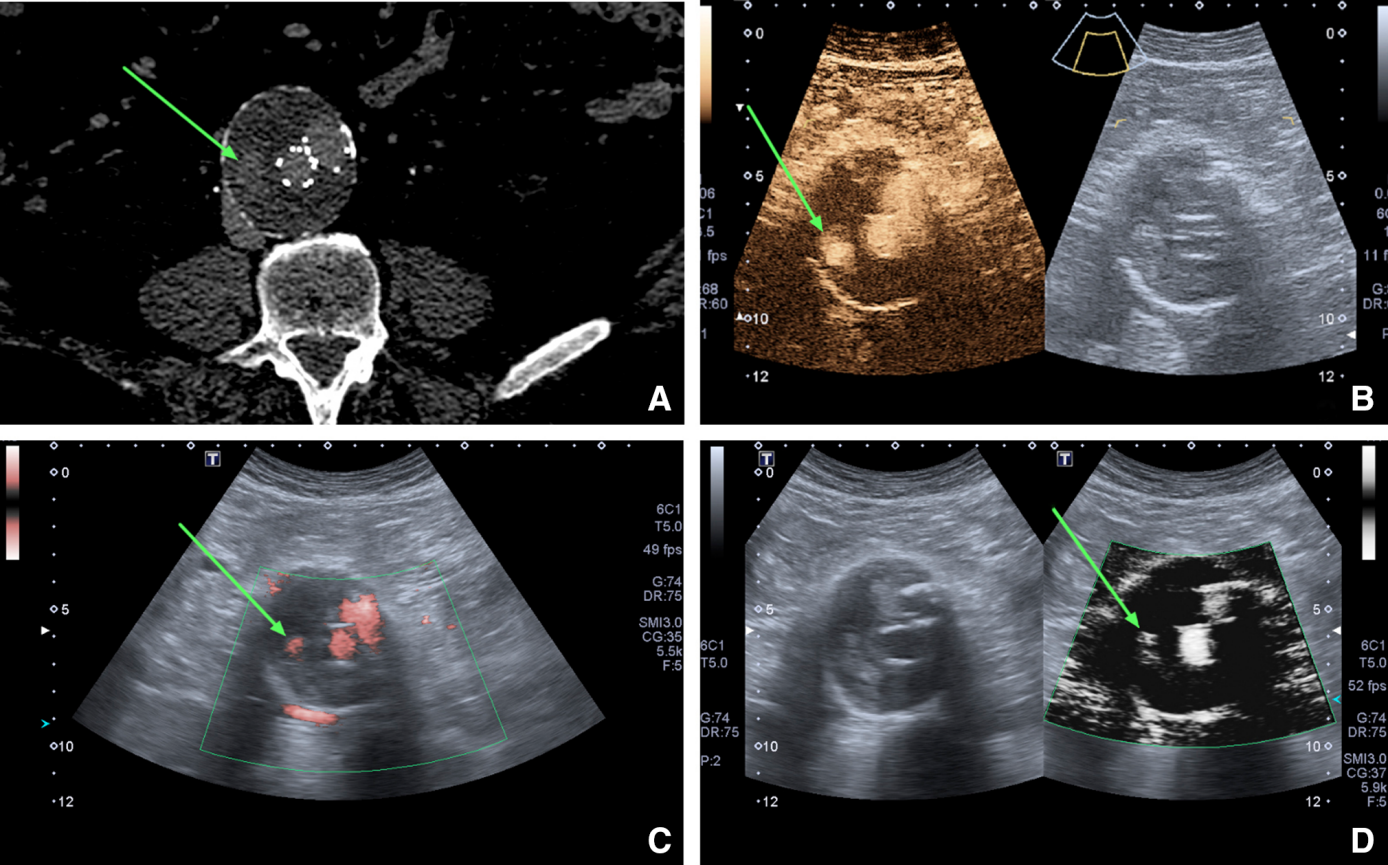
Cross section through an abdominal aortic aneurysm showing a type IIb endoleak (green arrow). A Computed Tomography Angiography, B Contrast-Enhanced Ultrasound, C color Superb Micro-vascular Imaging; D monochrome Superb Micro-vascular Imaging
